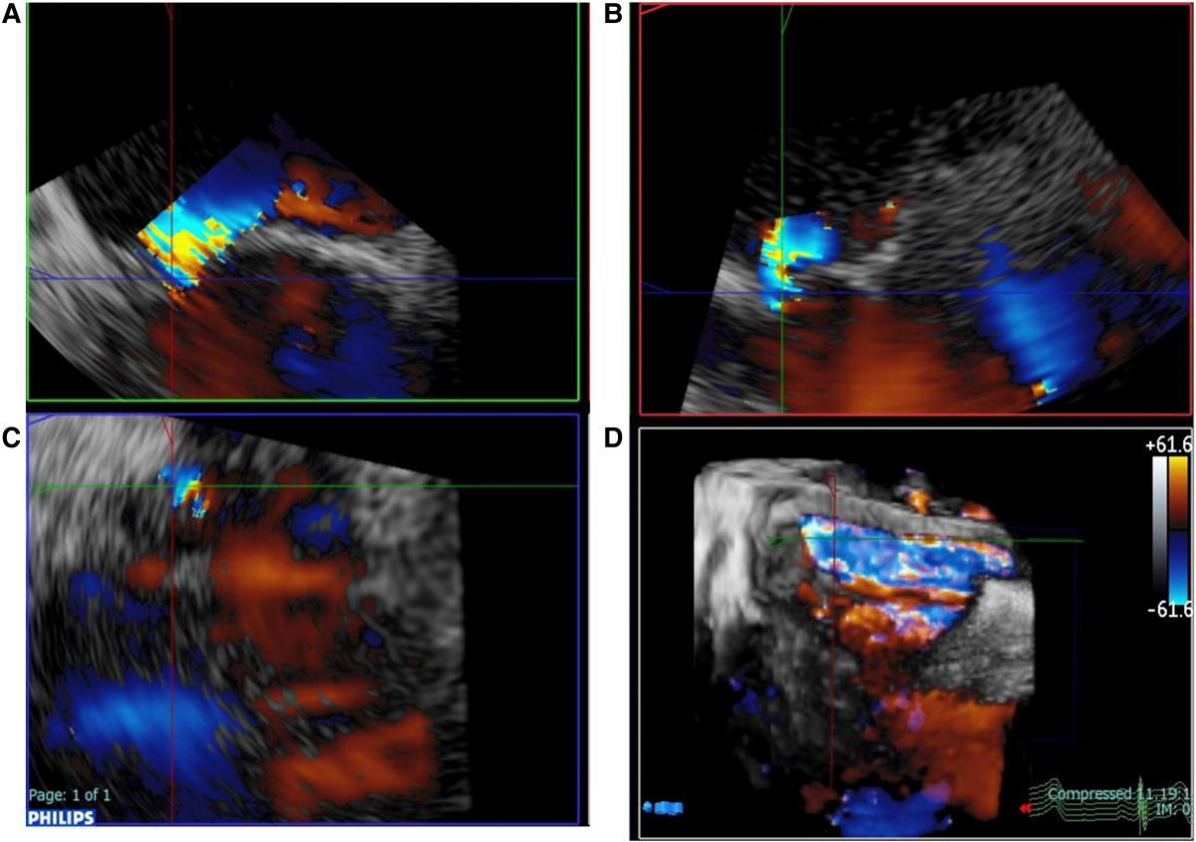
Second transoesophageal echocardiography. Commissure (*A*), long-axis (*B*), and short-axis (*C*) views reconstructed from three-dimensional image (*D*). Reconstructed images showed narrow mitral regurgitation jet through a pinhole perforation at the A3 segment.
